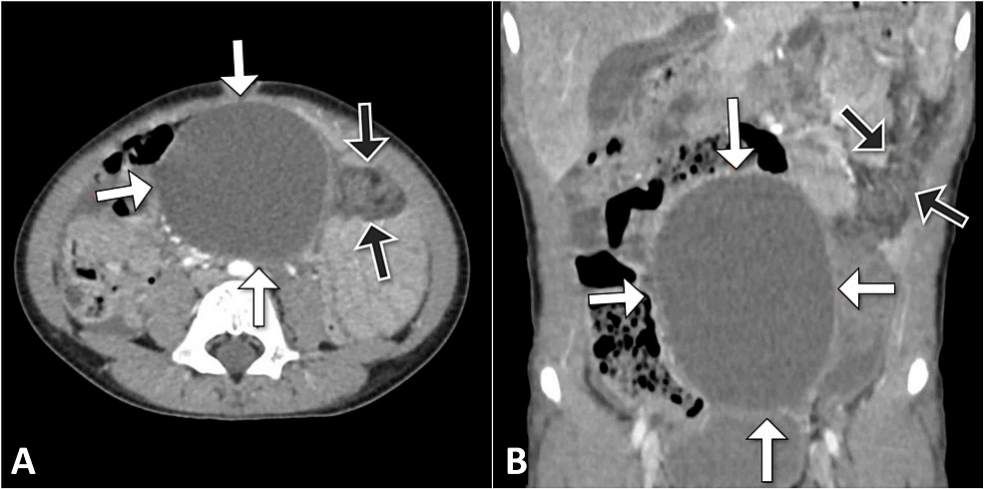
Axial (A) and coronal (B) CT images of the abdomen reveal a well-defined cystic lesion in the mid-abdomen (white arrow), accompanied by an adjacent area of fat density featuring distinctive streaks of whirling and concentric patterns (grey arrow). These radiological findings are indicative of an omental cyst with torsion CT: computed tomography
